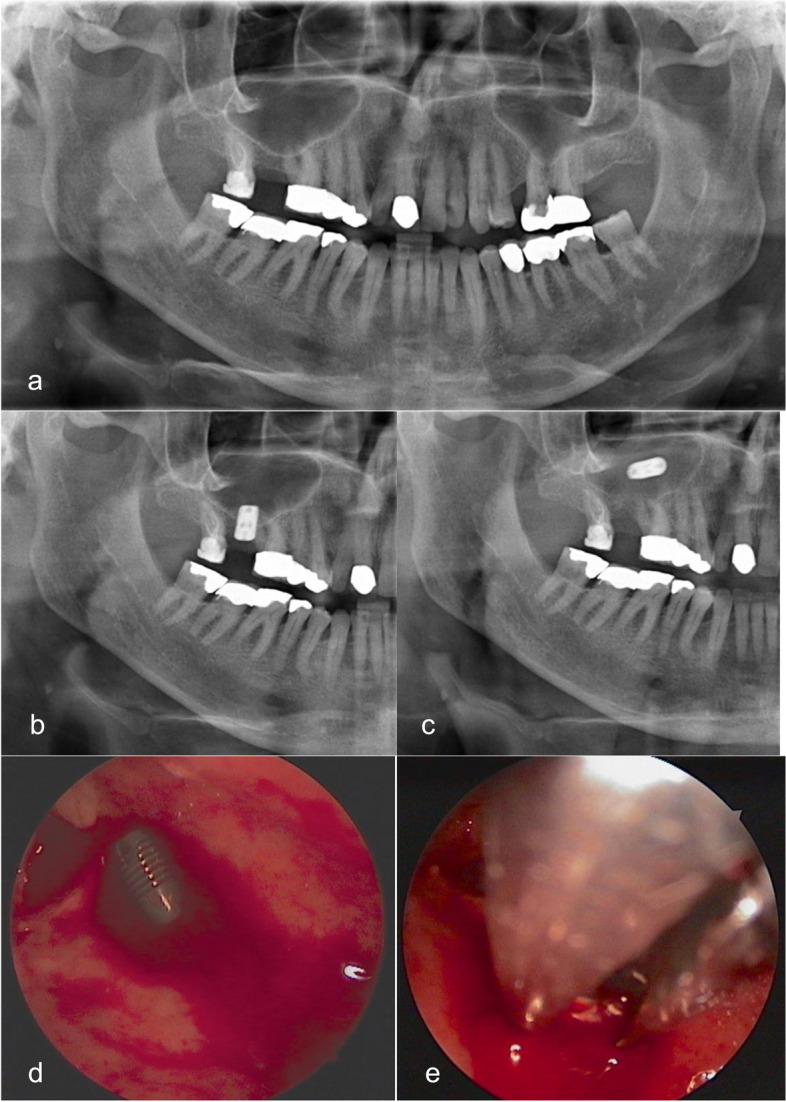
Case 1 - Implant displacement into maxillary sinus and immediate removal under local anaesthesia. a Baseline Panoramic radiograph showing limited vertical residual bone in the right upper second molar area. b Postoperative radiograph taken after completion of osteotome sinus floor elevation and implant insertion. c Panoramic radiograph taken immediately before removal procedure: shows implant migration into the right maxillary sinus following second-stage surgery four months later, along with mucosal thickening indicative of maxillary sinusitis. d Intraoperative endoscopic view of the displaced dental implant though the upper maxilla after creating a bony window and e Endoscopic-assisted removal of the implant using Blakesley forceps
